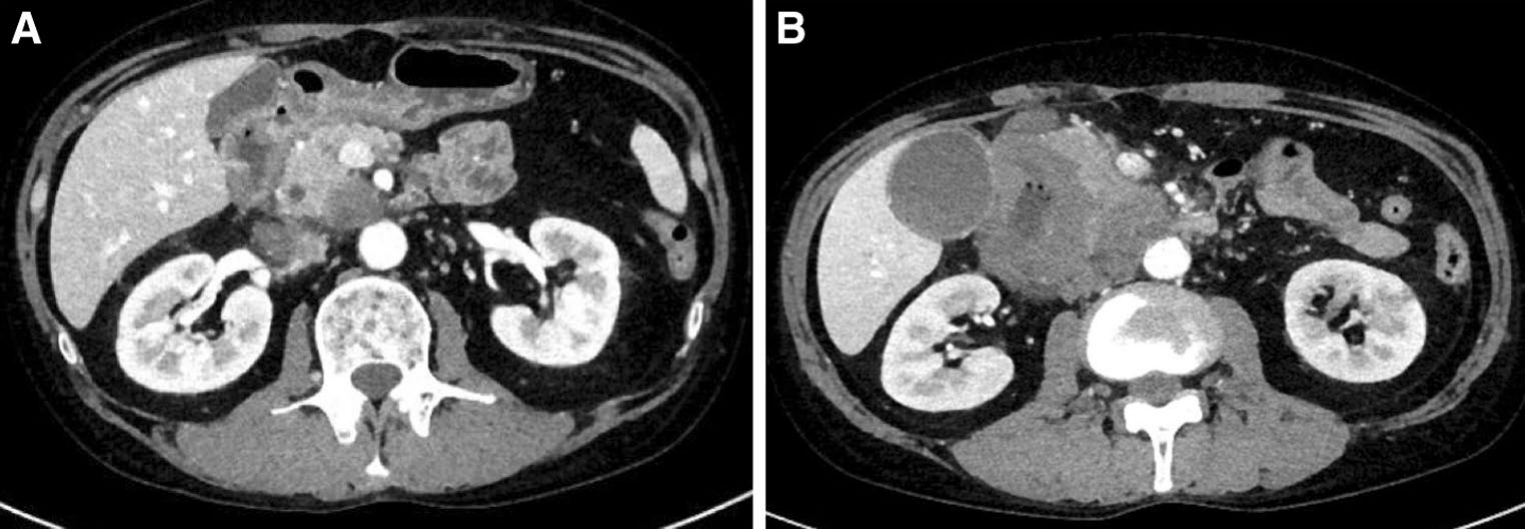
The CT scan showed pancreatic head swelling and slight peripancreatic fluid effusion (a). The enhanced CT scan showed an all-round wall thickening of the descending portion of the duodenum and the ill-defined mass lesion invading the pancreatic head (b)
